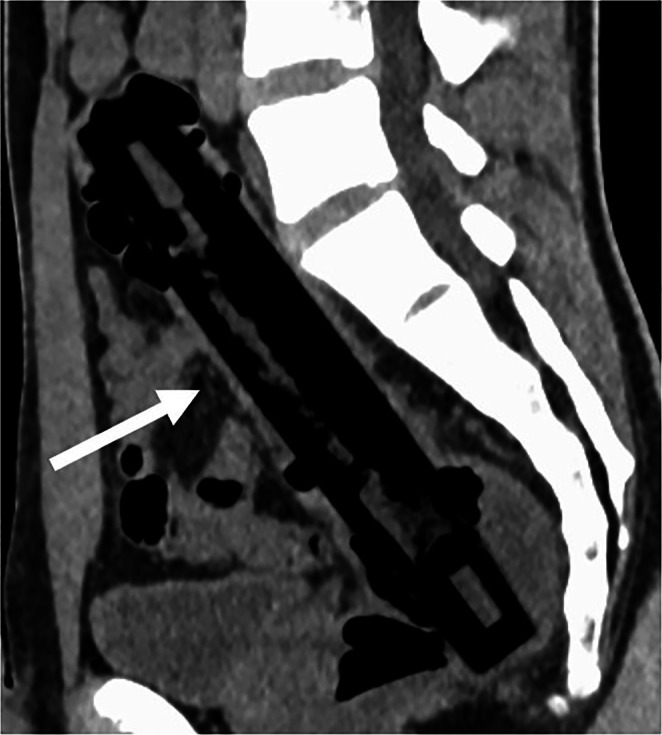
Sagittal CT demonstrating the long‐axis configuration of the object (arrow).
